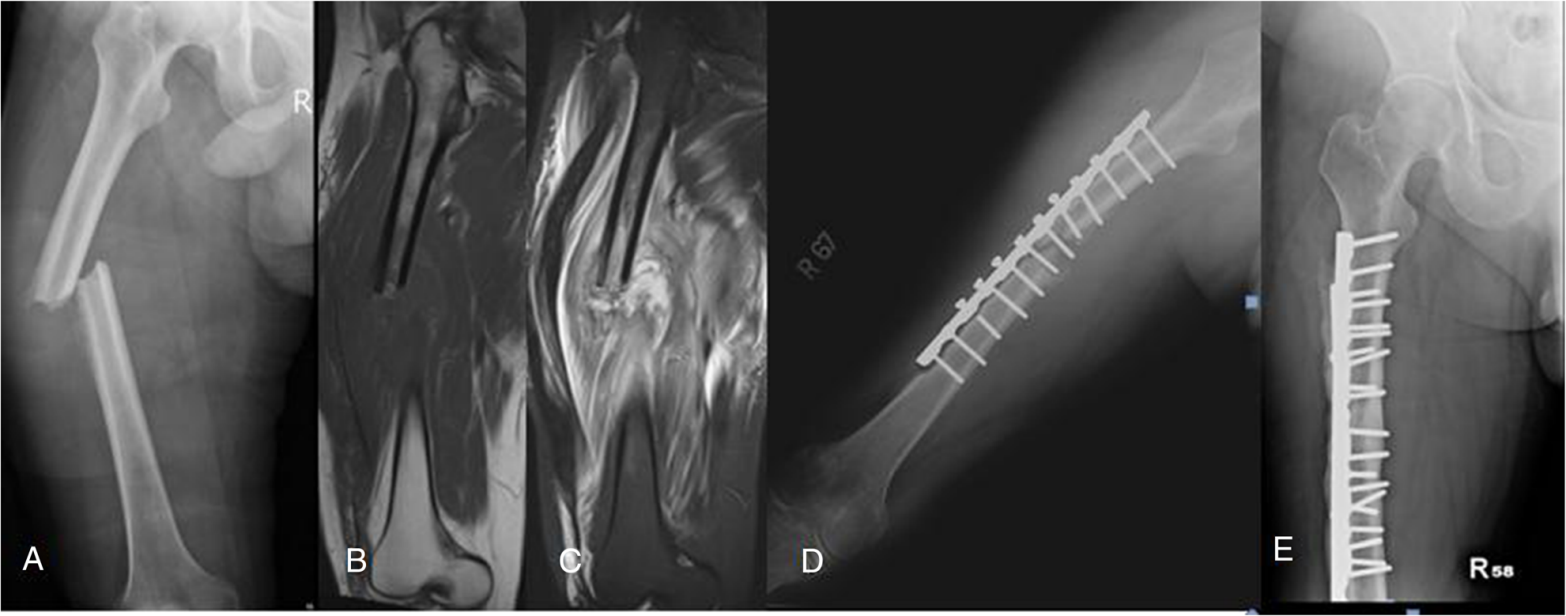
A 37-year-old man with a history of type 2 diabetes mellitus. (A) Plain film of the femur showing a pathological fracture with lateral cortex thinning and laminated periosteal reaction. (B, C) T1- and T2-weighted magnetic resonance imaging (MRI) showing severe soft tissue swelling and edematous change. (D) Plain film taken two months after open reduction and internal fixation (ORIF) and debridement surgery showing recurrent osteomyelitis with loss of reduction and laminated periosteal reaction of the medial cortex. (E) Plain film taken one year after revision debridement and ORIF surgery showing bony union without signs of osteomyelitis recurrence.
